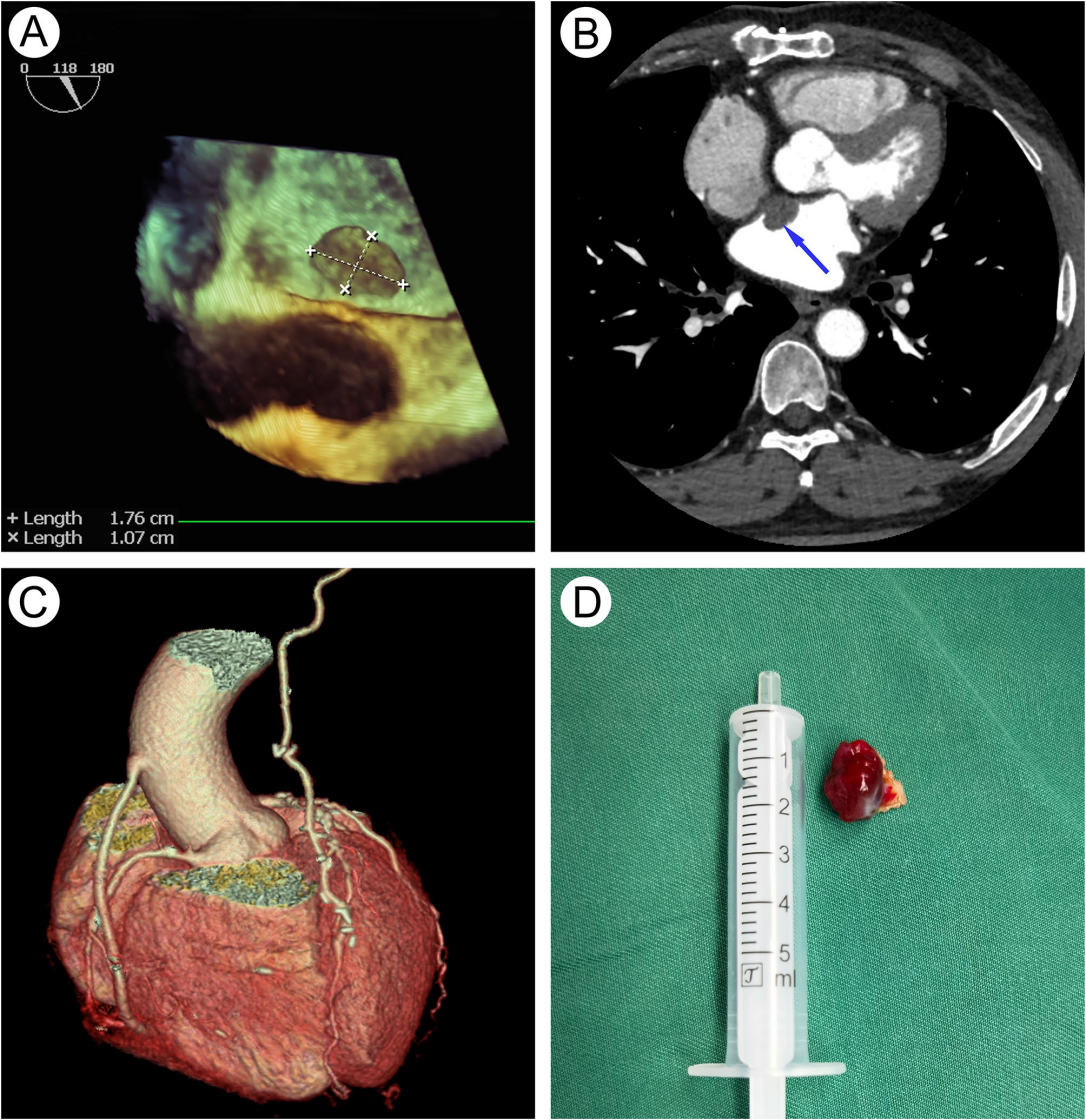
Excision of left atrial myxoma after coronary artery bypass grafting. a 3D transesophageal echocardiography revealed a hyperechoic lesion (1.76 cm × 1.07 cm) attached to the interatrial septum in the left atrium. b Computed tomography coronary angiography confirmed the mass with a low-density shadow. c The coronary artery bypass grafts of the saphenous vein graft and left internal mammary artery graft were smooth. d A pedunculated, jelly-like cardiac myxoma was completely resected
